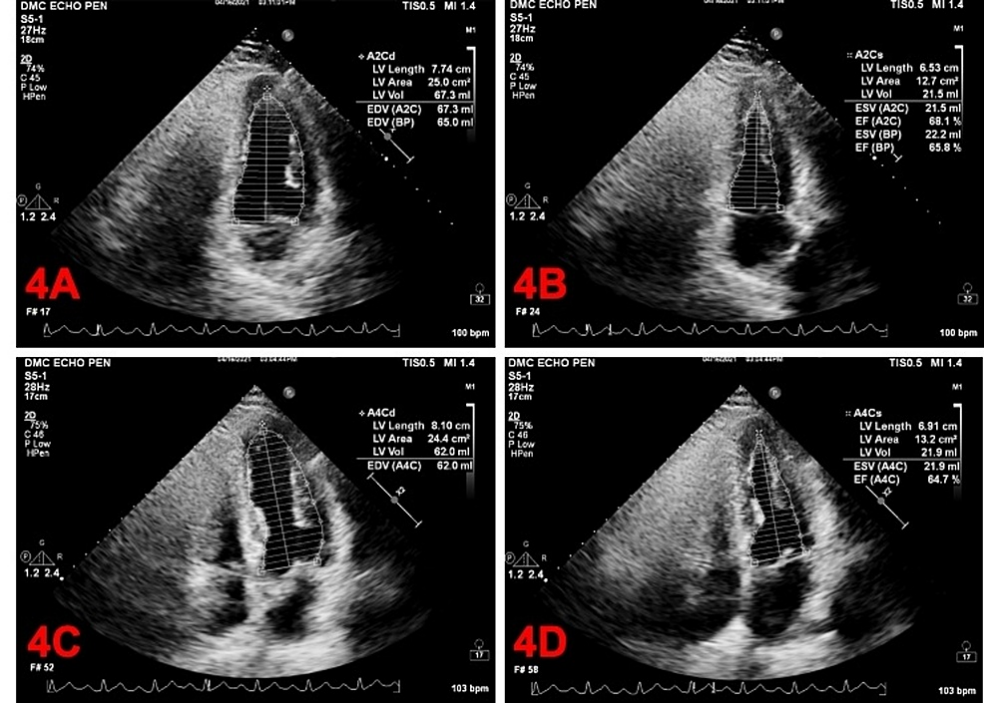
Modified Simpson method. A: Apical two-chamber diastole. B: Apical two-chamber systole. C: Apical four-chamber diastole. D: Apical four-chamber systole. A2C = apical two chamber; A2Cd = apical two chamber diastole; A2Cs = apical two chamber systole; A4Cd = apical four chamber diastole; A4Cs = apical four chamber systole; LV = left ventricular; BP = biplane; EDV = end diastolic volume; EF = ejection fraction; ESV = end systolic volume.
